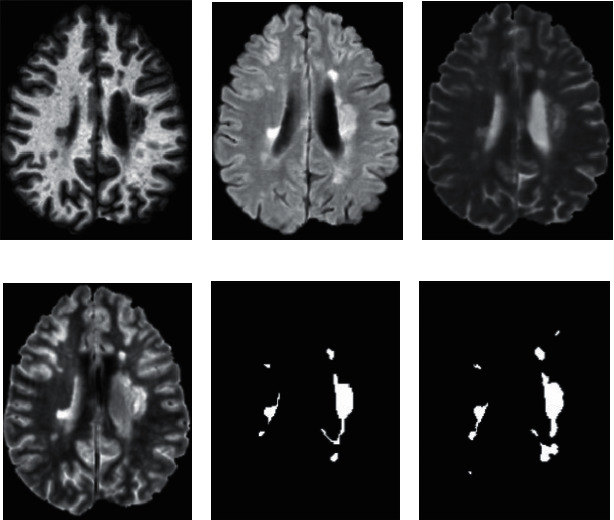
Sample of the ISBI dataset: (a) T1w, (b) FLAIR, (c) T2w, and (d) PDw, (e) manual delineation by rater 1, and (f) manual delineation by rater 2.
